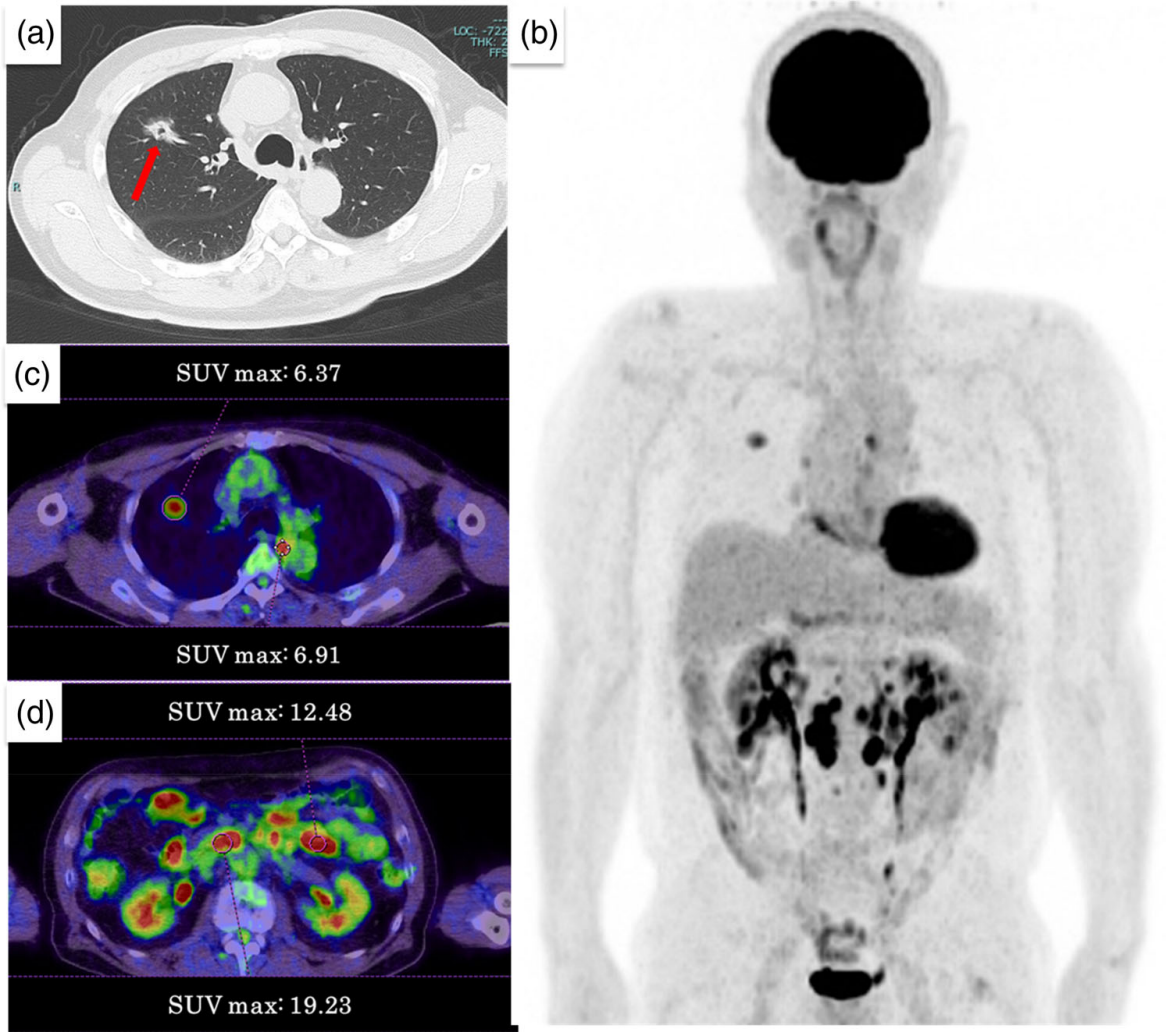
Computed tomography (CT) and fluorodeoxyglucose (FDG)‐positron emission tomography (PET)‐ CT image of the chest. (a) A plain CT scan of the chest showed a 27‐mm‐sized, part‐solitary, frosted shadow in the S3 of the right upper lobe (arrow). There was no evidence of enlarged hilar or mediastinal lymph nodes or pleural effusion. FDG‐PET showed FDG accumulation in the right lung mass, esophagus, and several intra‐abdominal lymph nodes (b). Maximum standardized uptake value (SUV) max was 6.37 for lung lesions (c), 6.91 for the esophagus (c), and 19.23 for the abdominal lymph nodes (d).
